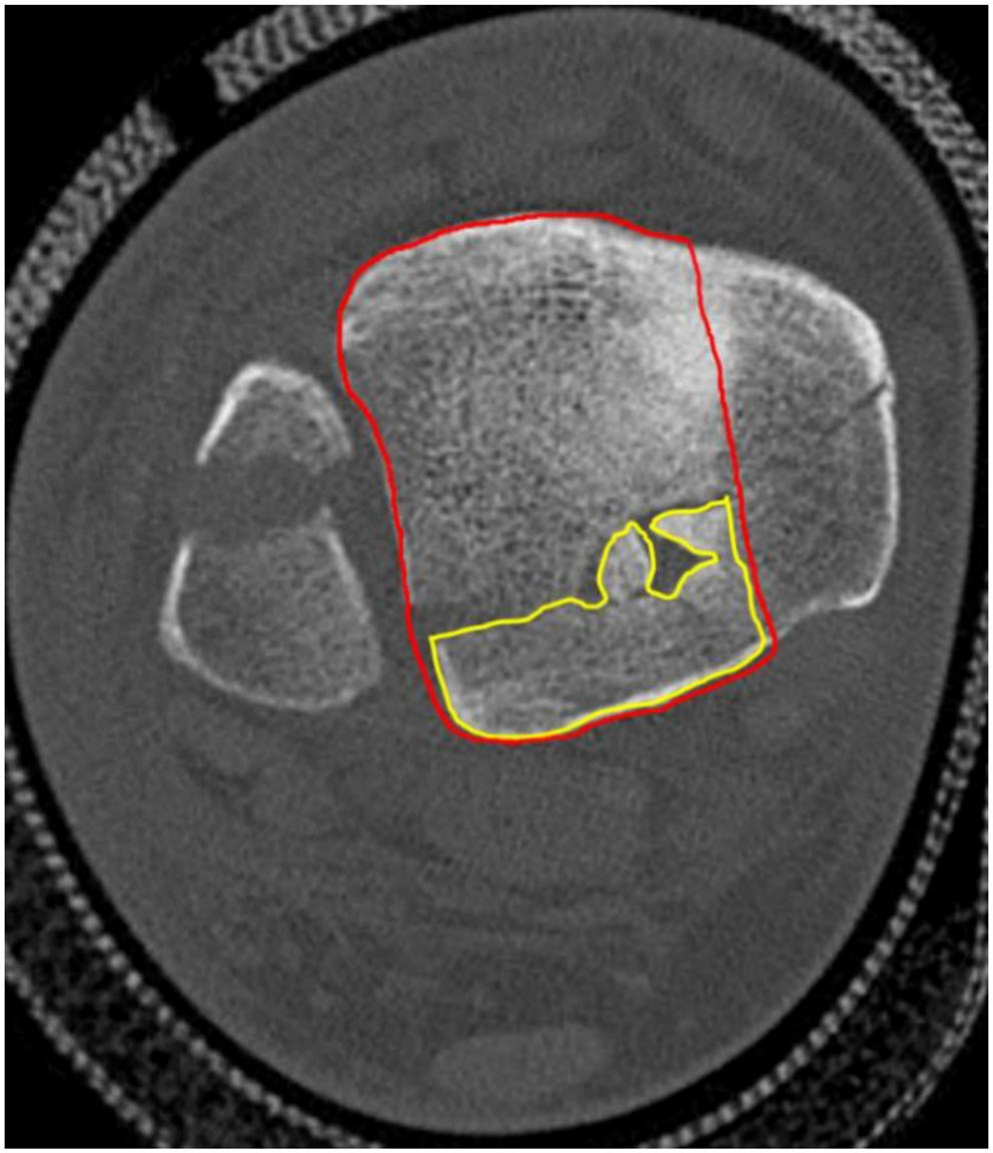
In the method 1, the PMF area is divided by the total articular surface of the tibia minus the medial malleolus on the axial view of the CT then expressed in %
